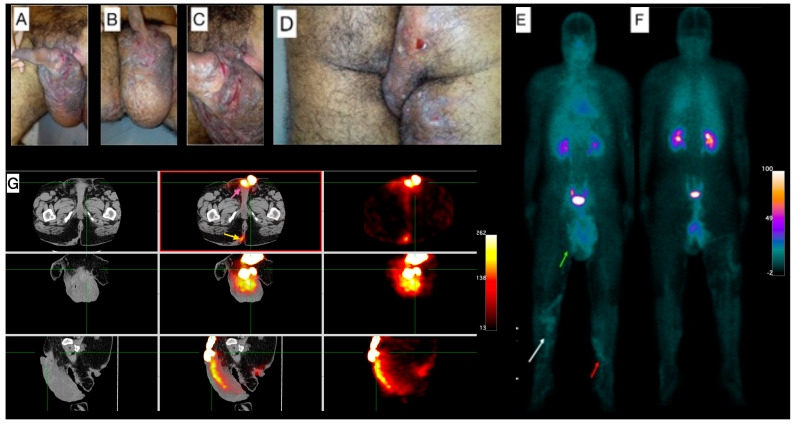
Patient 1 clinical and imaging findings. (A) External genitalia and inguinal region skin lesions. Noticeable lymphedema in the scrotum. (B) Lengthy fissures on the basis of the penis, and (C) lymphangiectasia on the scrotum’s skin. (D) Gluteal region shows pronounced edema, hyperchromia, fistulae, and lichenification, extending into the proximal third of the thighs. (E) Whole-body [^99m^Tc]Tc-anti-TNF-alpha scintigraphy, anterior view, demonstrates abnormal uptake in the scrotum (green arrow). A venous tract is visible in the distal thigh extending to the proximal third of the right inferior limb (white arrow) and the proximal third of the left lower limb (red arrow). (F) The posterior view of the scintigraphy further confirms abnormal uptake in the proximal third of the right thigh. (G) Tomographic images include a Computed Tomography (CT) scan on the left panel, fused Single-Photon Emission Computed Tomography/Computed Tomography (SPECT/CT) images in the center, and SPECT images on the right. These reveal abnormal uptake in the scrotum (pink arrow; top-center image) and the right intergluteal cleft (yellow arrow; bottom-center image).
